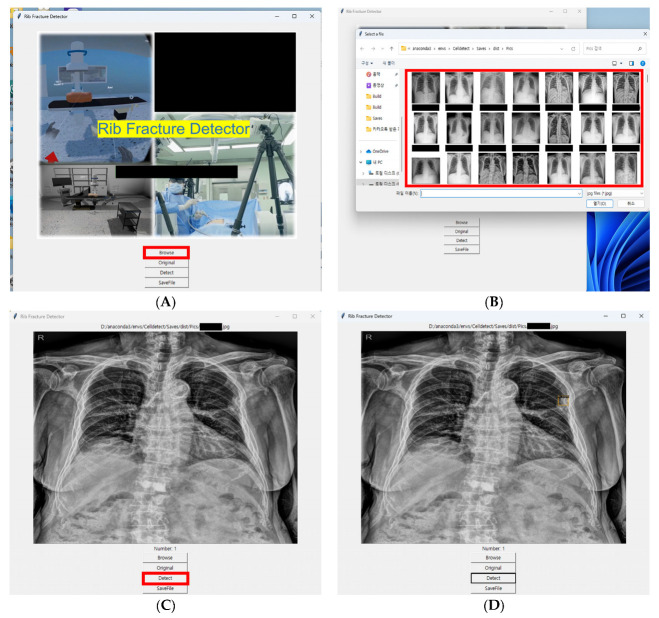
AI-powered rib fracture detection system interface and diagnostic output (A) The initial interface of the program is designed for convenient execution in a clinical setting. (B) A user-friendly interface is designed to facilitate easy selection of a file/image to be analyzed. (C) The original chest radiograph before the site of the fracture is identified. (D) The image with the site of the rib fracture identified and marked by the AI model. AI: Artificial Intelligence.
